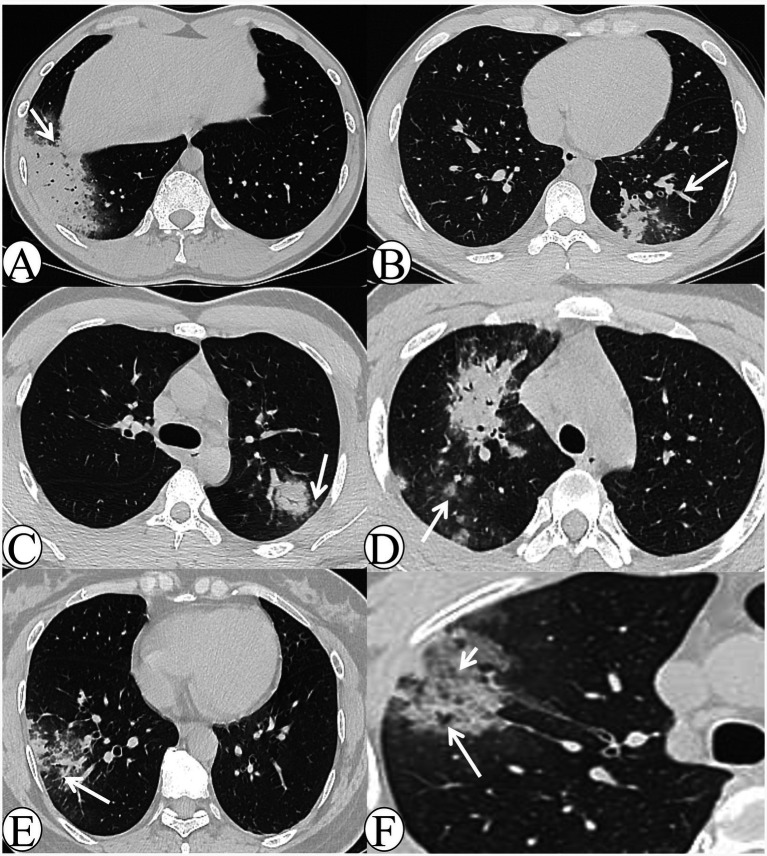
The CT images of early-stage *Chlamydia pneumoniae* pneumonia. (A) A 24-year-old male patient presented with fever, productive cough, and headache persisting for 5 days. Axial lung window CT demonstrates extensive consolidation in the right lower lobe with an air bronchogram (white arrow) and a visible peripheral halo sign. (B) A 21-year-old male patient presented with fever, pharyngeal hyperemia, and myalgia persisting for 6 days. Axial lung window CT demonstrates bronchial wall thickening in the left lower lobe, accompanied by patchy consolidation and ground-glass opacity (GGO), together with distal tree-in-bud and centrilobular nodules (white arrow). (C) A 21-year-old male patient presented with chills and fever for 1 day. Axial lung window CT demonstrates a round-like patch in the left upper lobe, accompanied by an air bronchogram and surrounding ill-defined centrilobular nodules (white arrow). (D) A 16-year-old male patient presented with cough and expectoration for 5 days. Axial lung window CT demonstrates multiple mass-like and nodular-patchy consolidations with a halo sign in the right upper lobe (white arrow). (E) A 45-year-old female patient presented with chills and fever for 5 days. Axial lung window CT demonstrates peribronchial patchy consolidation and ground-glass opacity (GGO) in the right lower lobe, with visible interlobular septal thickening and lobular sparing (white arrows). (F) A 29-year-old male patient presented with fever and cough for 4 days. Axial lung window CT demonstrates patchy consolidation and ground-glass opacity (GGO) in the right upper lobe, with visible intralobular septal thickening (white arrowhead) and a reversed tree-in-bud sign (white arrow).
